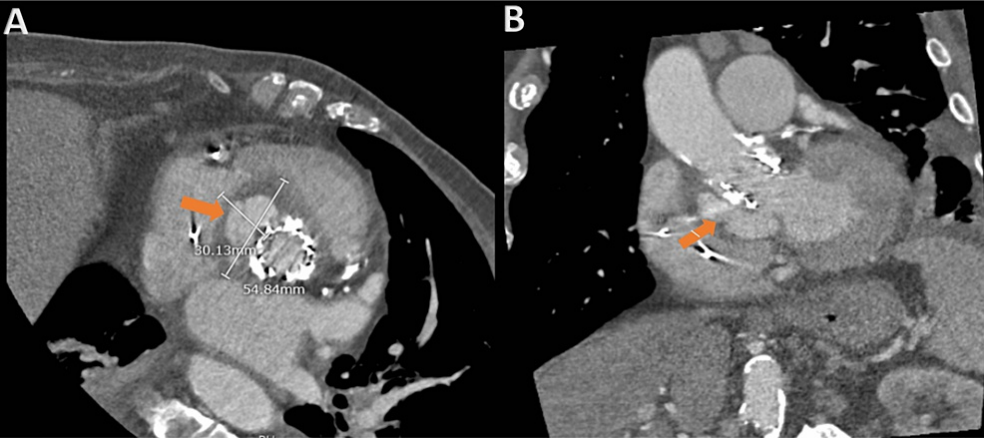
CT scan showing left ventricular pseudoaneurysm (arrows) on axial (A) and coronal (B) views
